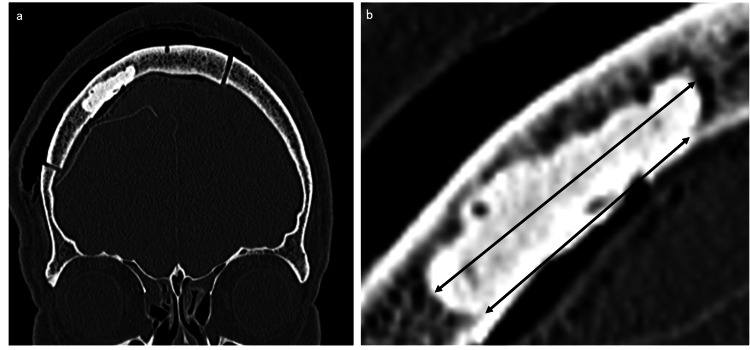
Postoperative CT To prevent the bone paste from falling off postoperatively, the skull bone was removed more widely on the diploic plate side than on the inner side (a: coronal imaging of postoperative CT, and b: enlarged view). The volume of bone defect was 3.77 cc (Ziostation2 version 2.9.5.1, Ziosoft, Tokyo).
